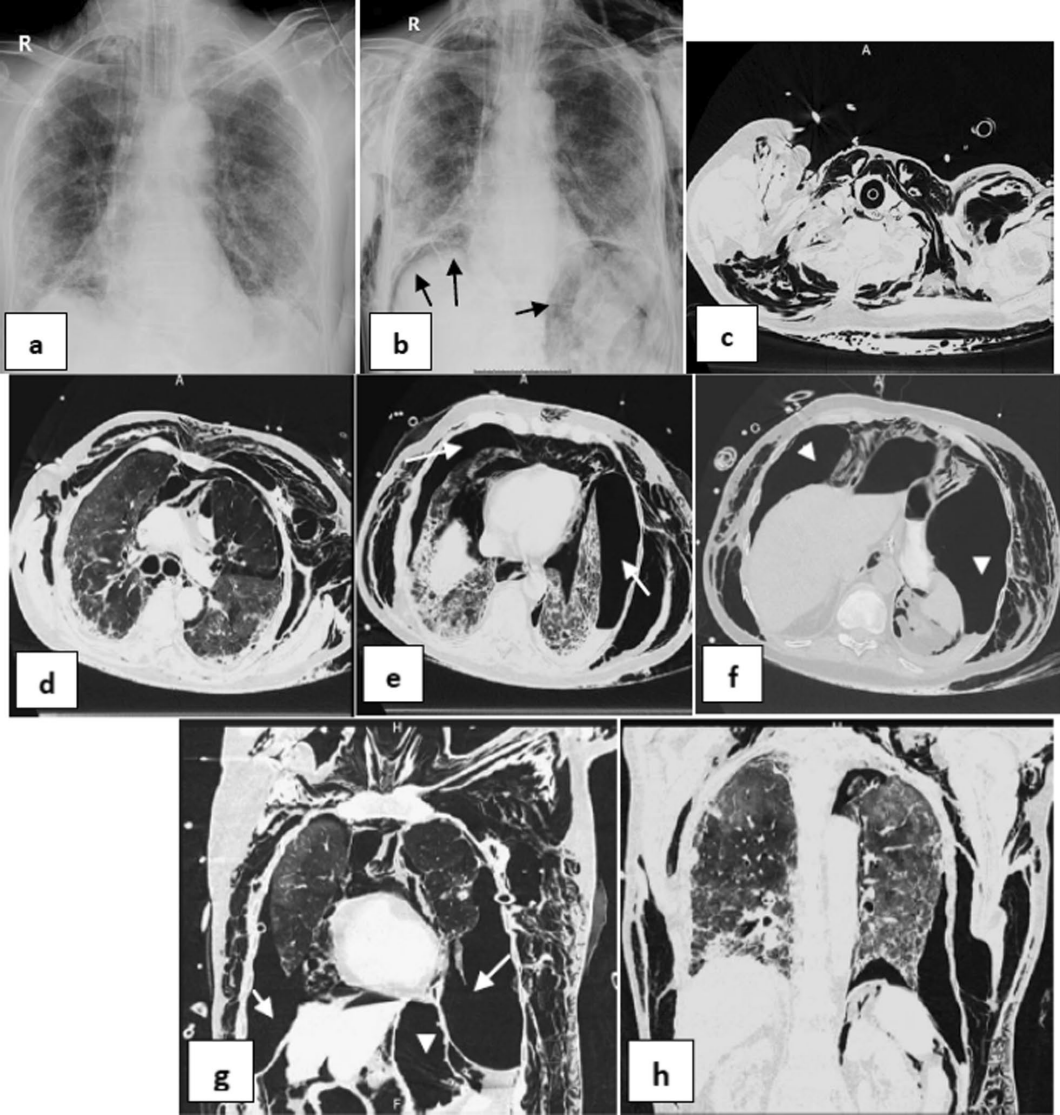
70-year-old male intubated 5 days after admission. a, b Two frontal chest radiographs depict moderate pneumperitonium (black arrows) and subcutaneous emphysema. Note bilateral diffuse mainly lower zones bilaterally ill-defined air space opacities with peripheral predominance. c–f series axial CT cuts, g, h coronal reconstructing images. Four days later, he developed large bilateral pneumothoraces (white arrows), extensive subcutaneous emphysema and moderate pneumoperitonium (white arrow heads)
